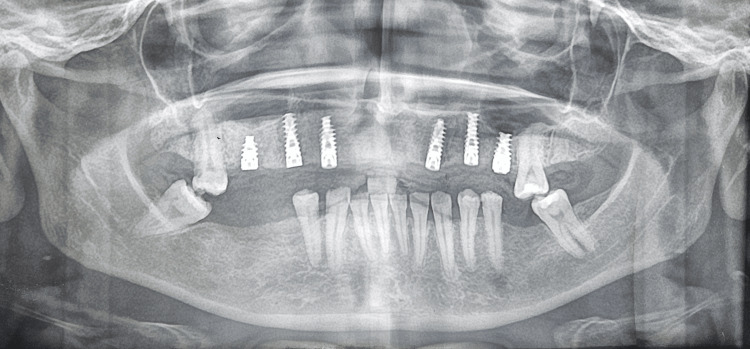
Postoperative orthopantomogram (OPG) OPG after implant placement.
